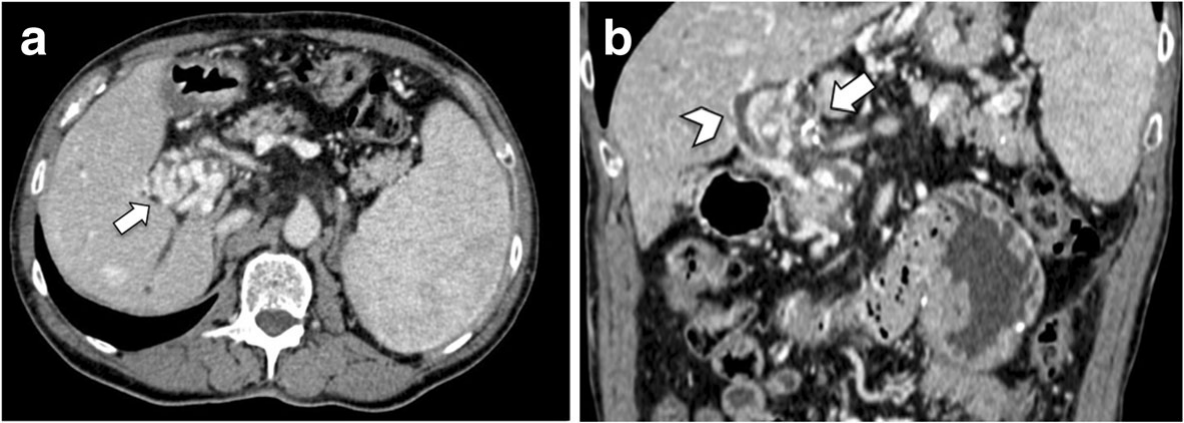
A 63-year-old woman with portal biliopathy. a Axial enhanced CT image shows the cavernous transformation of the portal vein (arrow). b Coronal CT image demonstrates biliary ducts dilatation (arrowhead) due to compression by portal cavernoma (arrow)
